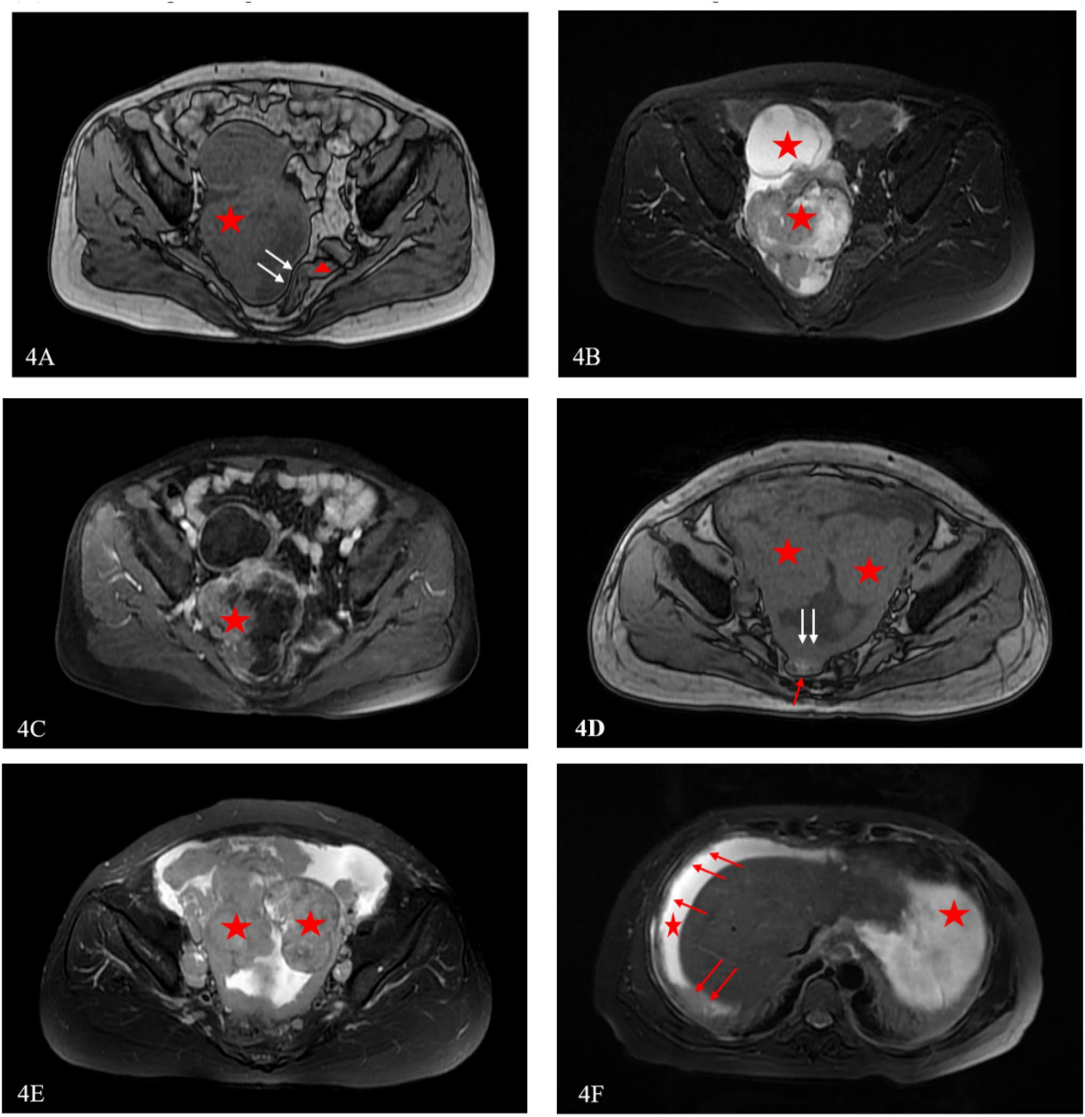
Two cases of MR images representing R0 (A–C) and R2 (D–F), respectively. A–C A 58-year-old woman with high grade SOC (HGSOC), who achieved R0 resection by PDS. A Axial T1DEI image shows a mass on right ovary (red star) without invasion (white arrows) of the adjacent rectum (red triangle). B Axial T2FSE image shows a cystic-solid mass on the right ovary (red stars). C Axial T1CE image shows the solid component of the right ovarian mass was significantly enhanced (red star). D–F A 49-year-old woman with HGSOC, who achieved R2 resection by PDS. D Axial T1-DEI image shows solid mass (red stars) of bilateral ovary. The metastasis implanted on pelvic peritoneal in front of rectum (red arrow) was fused to the rectum as a block (white arrows). E Axial T2FSE image shows solid mass (red stars) of bilateral ovary. F Axial T2FSE image shows large amount of ascites (red stars), and metastasis implanted on the diaphragm (red arrows)
